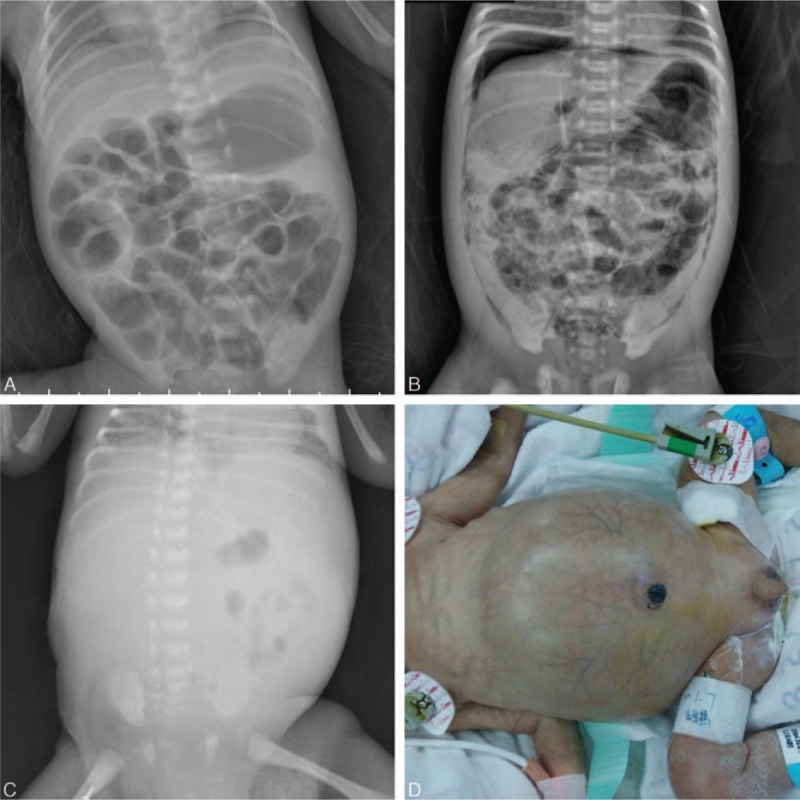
Radiographic findings of necrotizing enterocolitis. (A) Portal venous gas and pneumatosis intestinalis; (B) pneumoperitoneum and pneumatosis intestinalis; (C) gasless abdomen. Panel D shows an infant with distended abdomen with periumbilical erythema.
